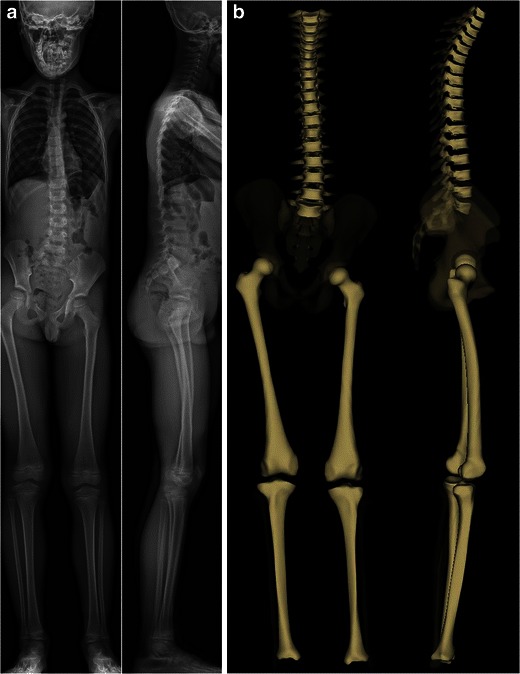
Radiographs of the spine and lower limbs in a 7-year-old boy. AP and lateral images are simultaneously obtained (a), allowing for surface 3D reconstructions (b). Relationships between the spine and the lower limbs can be assessed. [Total cumulative DAP = 584 mGy·cm^2^]
